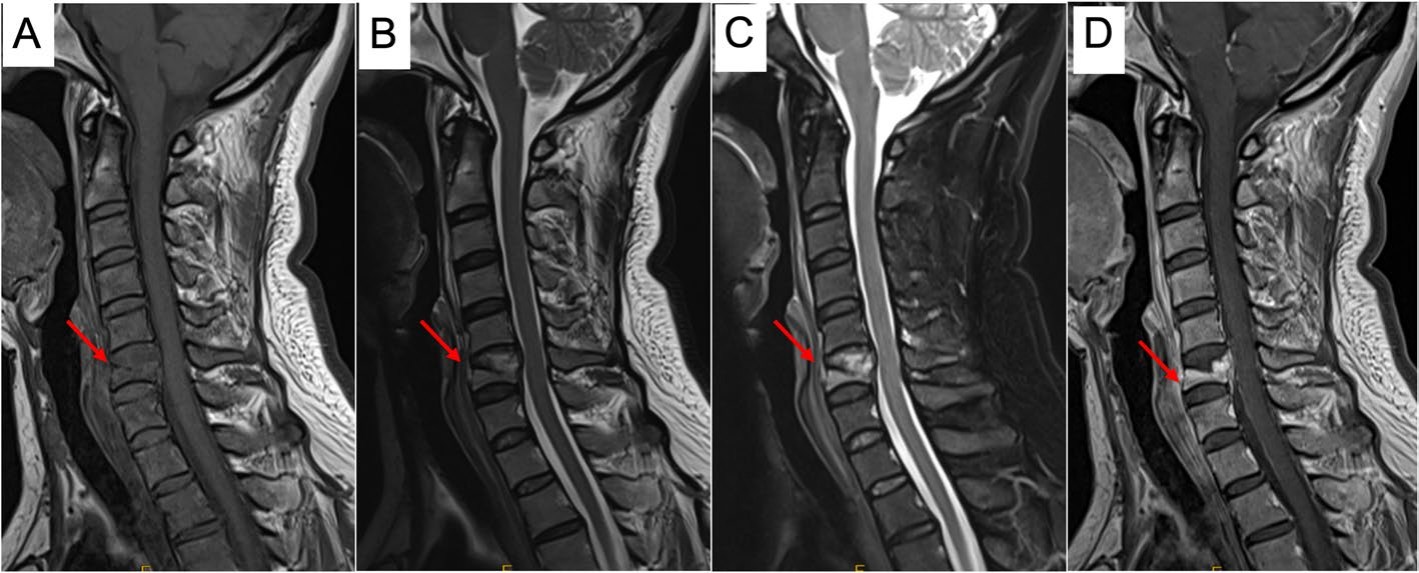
MRI of the cervical spine with contrast (gadolinium), sagittal cut images. A T1-weighted image; B T2-weighted image; C T2-weighted image with fat suppression; D Post-contrast T1-weighted image; arrows: C6 vertebral body
